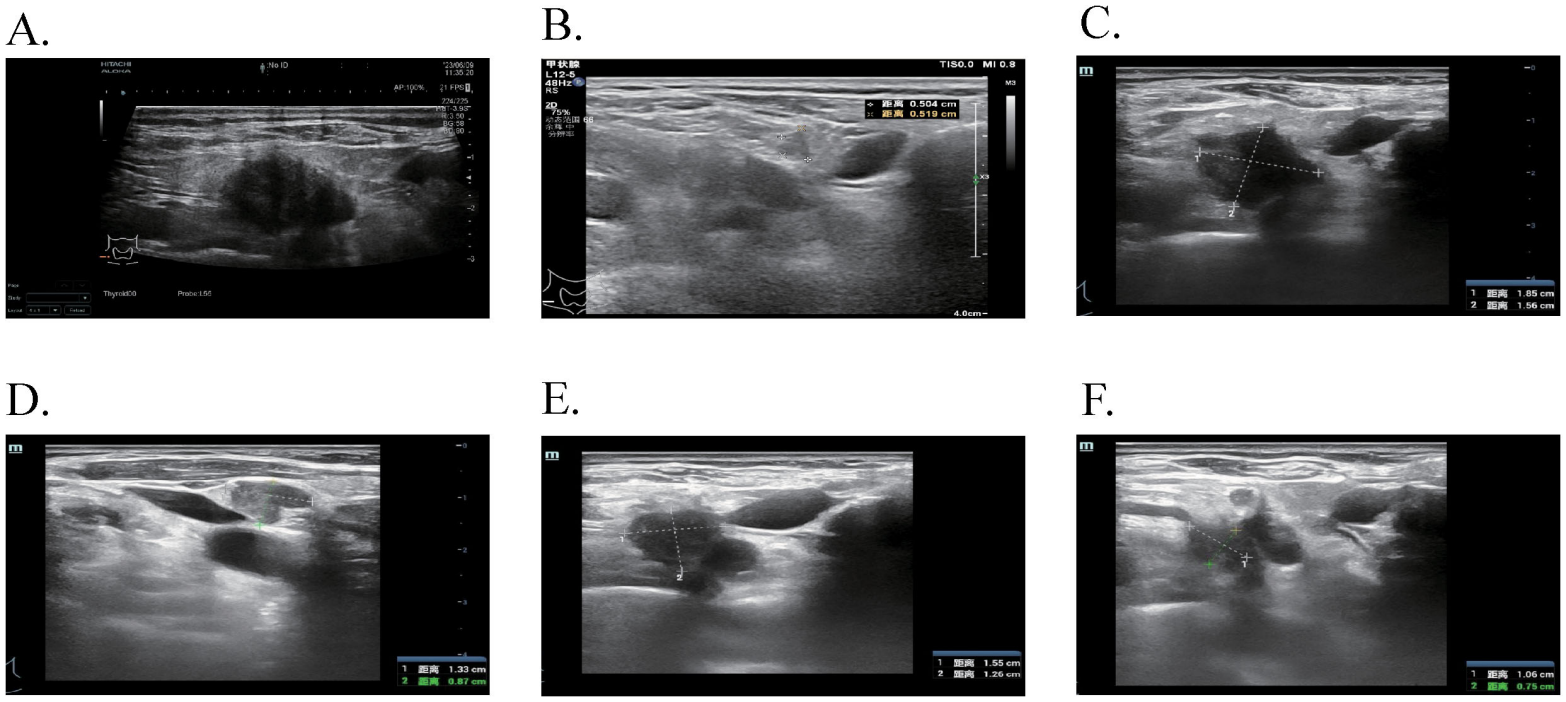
The ultrasound features of cervical lymph nodes at different time points. (A) After 24 months of Osimertinib, enlarged lymph nodes were observed in the IV region of the right neck, with a maximum size of 2.3 × 1.7 cm. (B) Following dual-targeted therapy, a previously enlarged lymph node in the IV region of the right neck reduced to 0.5 × 0.5 cm. (C, D) After 2 cycles of EP chemotherapy plus Adebrelimab, increased and enlarged lymph nodes were detected in the right neck IV area, with the largest measuring 1.9 × 1.6 cm and 1.3 × 0.9 cm. (E, F) With the addition of Osimertinib to the existing chemotherapy and immunotherapy regimen, the enlarged lymph nodes in the right neck IV area measured 1.6 × 1.3 cm and 1.1 × 0.8 cm.
